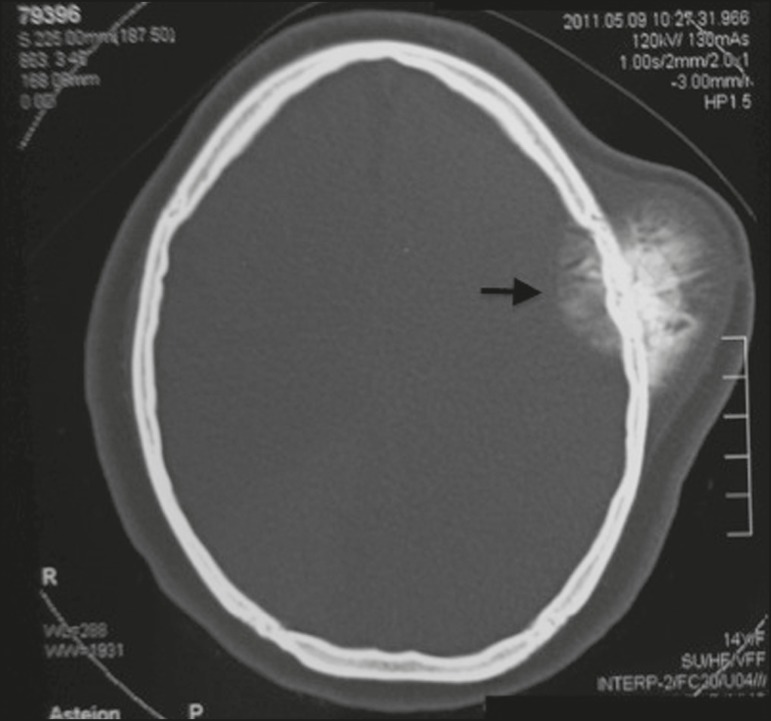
Computed tomography of the skull, showing a hyperintense
